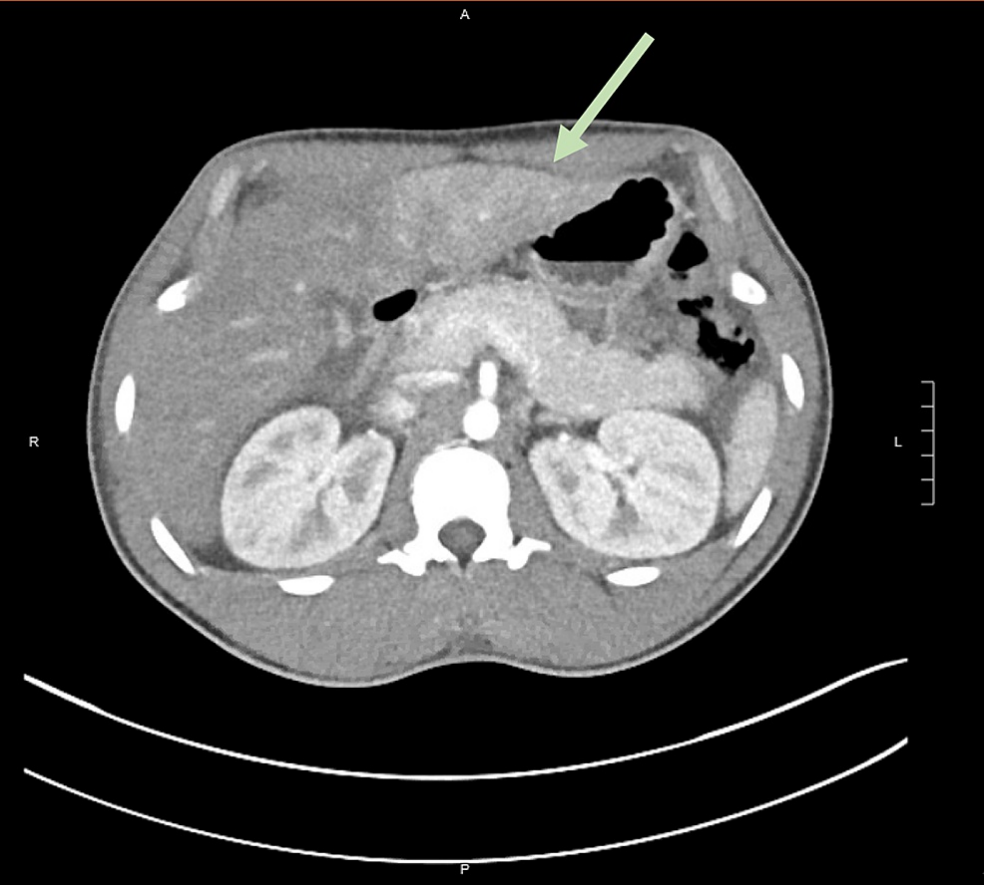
Abdominal CT scan with contrast in the arterial phase showing slight heterogeneous left lateral liver lobe enhancement (green arrow). Overall, the liver is normal in morphology and contour. CT: computed tomography
